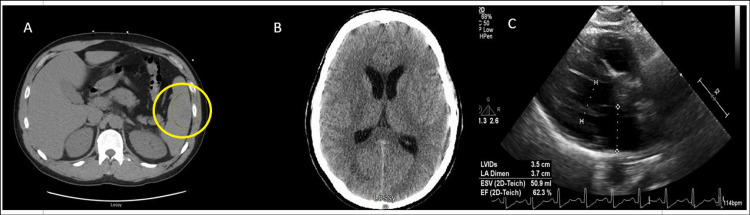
CT of the abdomen and pelvis showing mild hepatomegaly, mild splenomegaly, and possible splenic infarcts (A, yellow circle). CT of the head without contrast showing no acute intracranial process (B). TTE with a normal ejection fraction of 55%, no valvular vegetation, and BAV with mild stenosis (C). CT: computed tomography; TTE: transthoracic echocardiogram; BAV: bicuspid aortic valve
